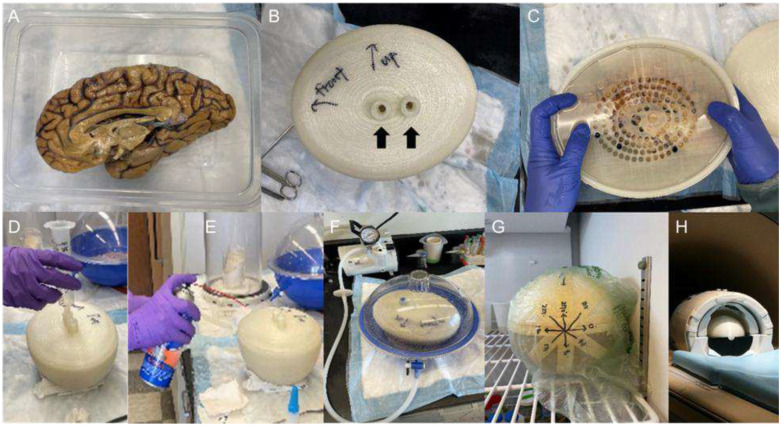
Procedures of brain sample preparation from formalin fixation to MRI scanning (A) The brain sample was transferred to phosphate-buffered saline with 2 mM gadopentetate dimeglumine two days before postmortem MRI scans. (B) The orientation of the brain in the container was marked on the outer lid. Black arrows indicate the top holes through which Fomblin^™^ was poured to the container. (C) The inner lid with plastic pins (black) and pieces was attached to the container to keep the brain sample stable during the MRI scans. (D) Using a 100 ml syringe, Fomblin^™^ was introduced through one of the top holes to fill the container to approximately 80%. (E) The outer lid was pushed to fit the bottom piece with super glue (Gorilla^™^) applied on the contacting surfaces. Spray adhesives (Permatex^™^) were then applied around the container to prevent leakage. (F) The container was placed into a vacuum chamber for more than 12 h, and then Fomblin^™^ was gently added through both holes on the outer lid to fully fill the container. (G) For the multi-orientation acquisition, the brain orientation and the degree of rotations were marked on the container, which was kept in the 35°F refrigerator until the MRI scans. (H) The brain sample was transported to the MRI facility, placed in the head coil, and scanned using the desired MRI pulse sequences.
